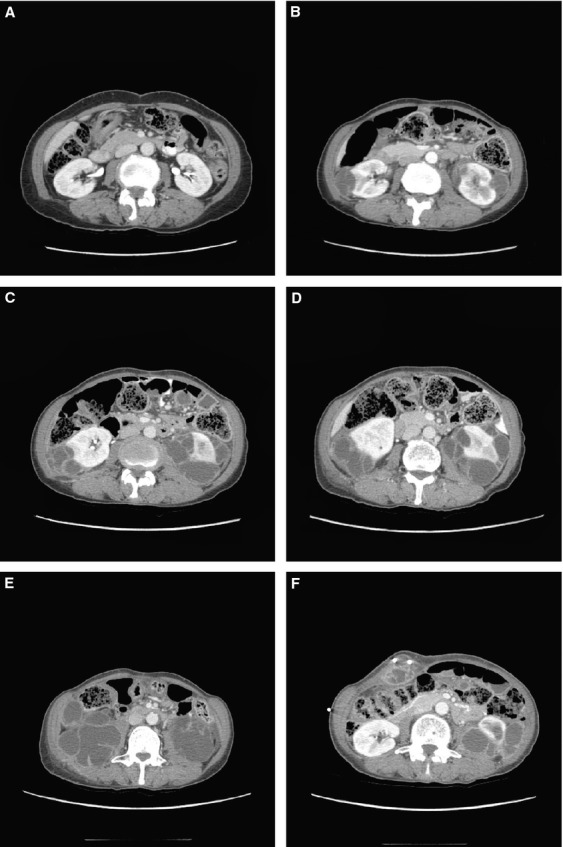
Computed tomography images of a 61-year-old woman with *ALK*-positive NSCLC who developed complex renal cysts during treatment with crizotinib (patient 1, Table 1). (A) baseline and (B) 2.5 months, (C) 4.1 months, (D) 5.5 months, (E) 6.3 months (just prior to cyst resection), and (F) 18.1 months after initiation of treatment. The images show progressive growth of multiseptated renal cystic lesions infiltrating the perirenal space and involving the left psoas muscle. Additionally, a cystic lesion in the anterior abdominal wall developed (drain in place, F), which was resected. Pathology showed degenerated muscular tissue with fibrosis and acute inflammation. NSCLC, non–small cell lung cancer. ALK, anaplastic lymphoma kinase.
